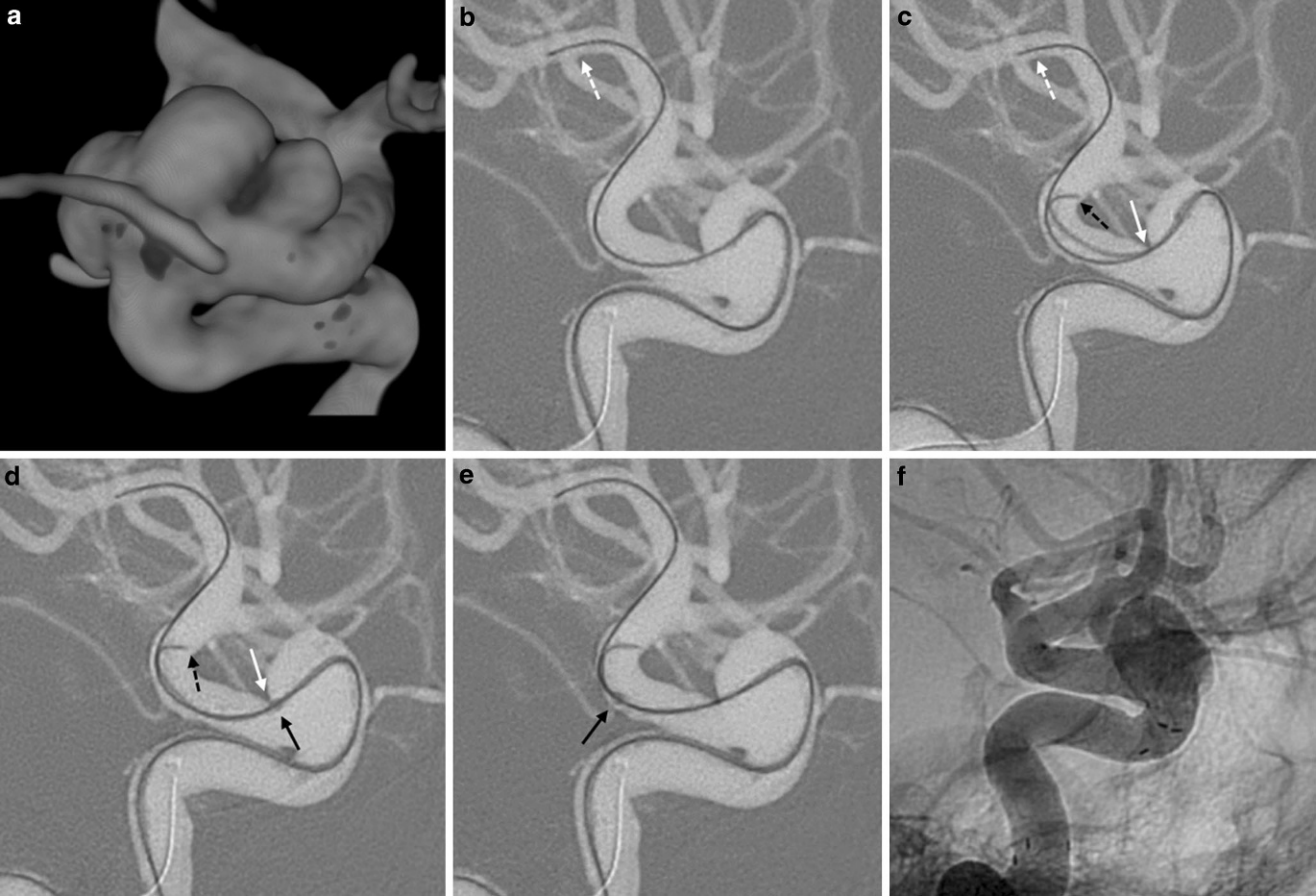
Female patient with an incidental right paraophthalmic ICA aneurysm. a Periinterventional planning 3D-rotational angiogram demonstrated the wide-necked, trilobulated morphology of the aneurysm. b A Synchro 0.014in. microwire (*white dashed arrow*) was navigated across the aneurysm. c–e Due to the lacking support of a single microwire and stiffness of the XT-27 microcatheter the catheter bulged into the aneurysm sac, and due to the off-set between the microwire and the tip of the microcatheter (*black arrow*) was stuck on the ledge (*white arrow*) between the aneurysm neck and the parent artery preventing to be advanced distally. A Transend 0.010in. microwire (*black dashed arrow*) was then introduced into the XT-27 in parallel to the Synchro 0.014in providing more support for the microcatheter to be advanced past the aneurysm and positioned distally. A Surpass Evolve (Stryker Neurovascular, Fremont, CA, USA) was subsequently deployed. The cone beam CT (not shown) demonstrated some fish-mouthing at the proximal end of the FD. f A Neuroform Atlas stent was then deployed to improve wall apposition in the proximal part of the FD
